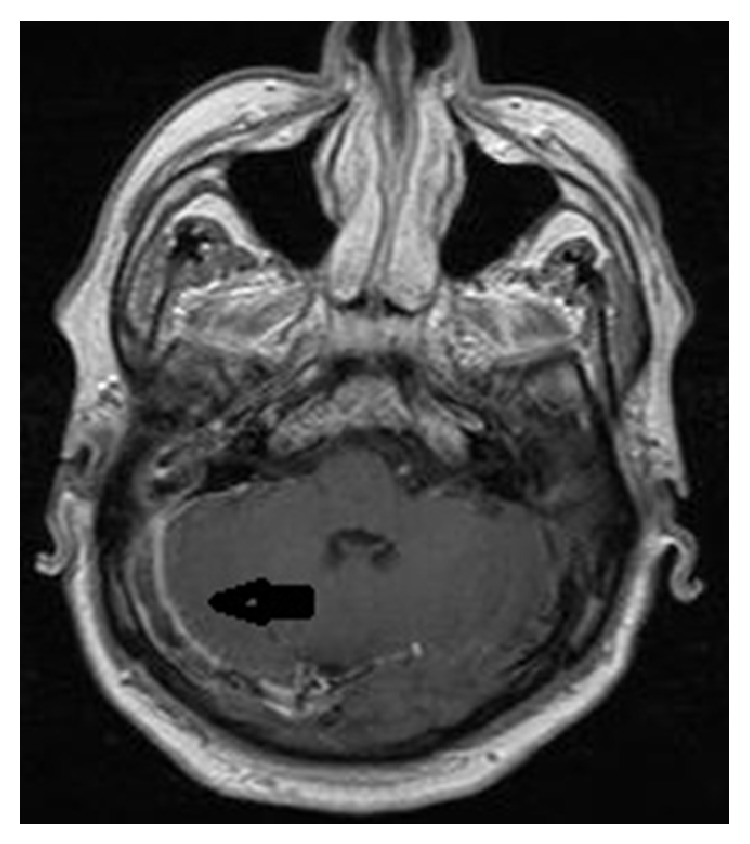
Axial brain MRI with contrast showed filling defects in right sigmoid and transverse sinuses.
